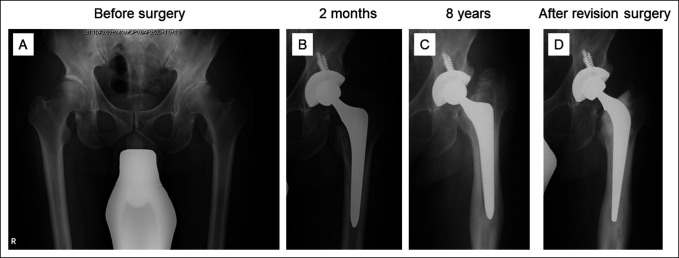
A, Radiographs showing a 57-year-old man presented with right hip pain caused by severe osteoarthritis secondary to dysplasia. B, He underwent primary total hip arthroplasty using an Elance stem. C, Radiograph obtained at 8 years after surgery. A massive stem subsidence of 27.8 mm was observed. D, The patient underwent an isolated stem revision using a cemented stem. The stem was manually mobilized and was easily removed from the femoral canal with a hammer. No signs of infection such as abnormal fluid collection were observed during the surgery.
